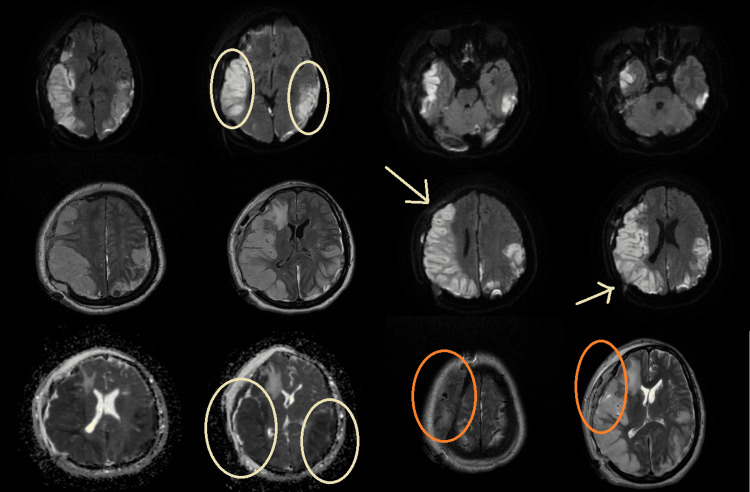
Significant ischemic infarction in the right hemisphere with hemorrhage and mineralization on MRI.
